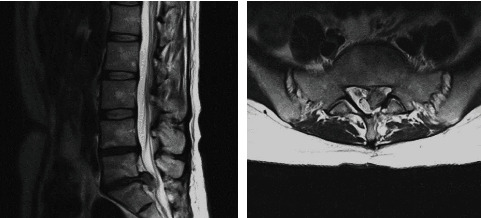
Intervertebral disc extrusion visible in the MRI.
